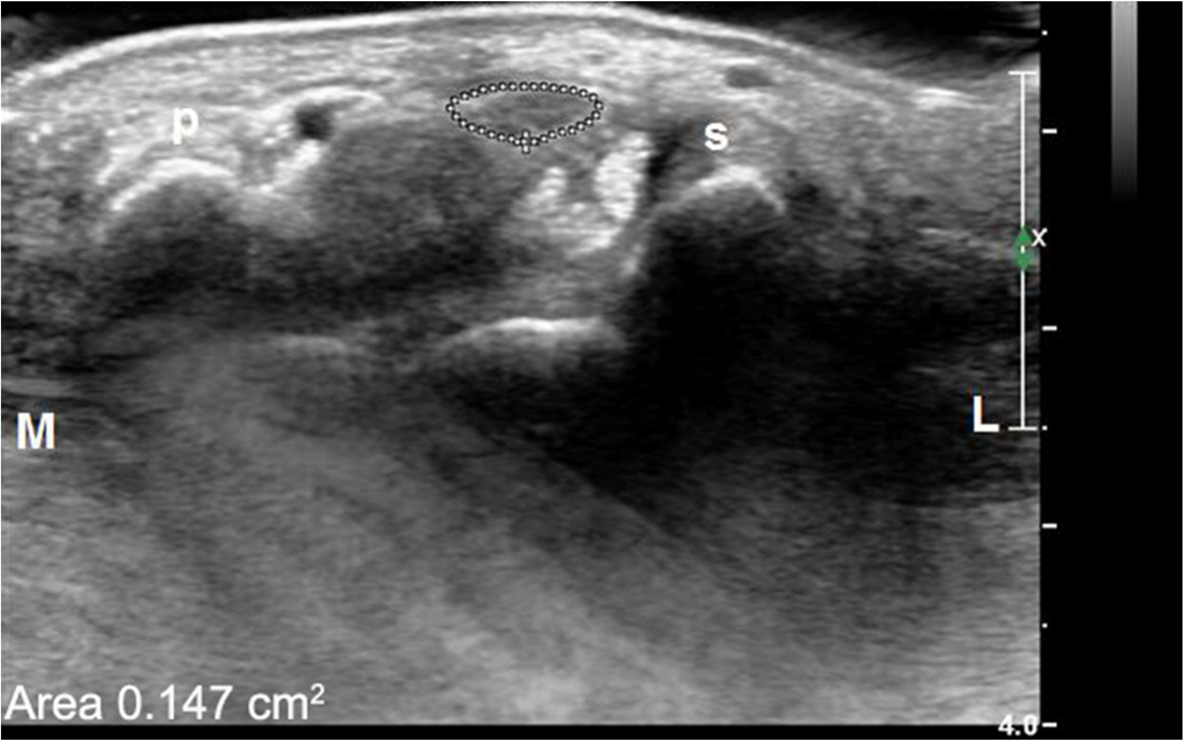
Transverse ultrasonic image of the proximal carpal tunnel at the level of the pisiform bone showed thickened median nerve and reduced echo. The area of the median nerve was 0.147 cm^2^. *S* scaphoid bone; *P* pisiform bone; *L* lateral; *M* medial
